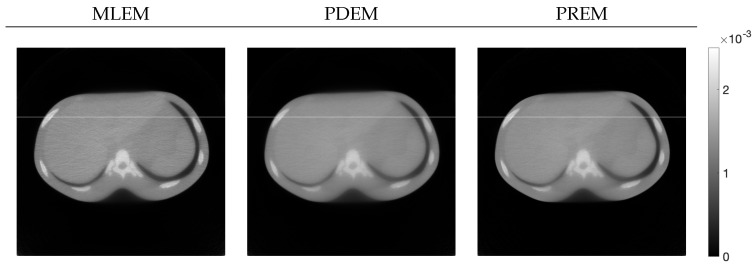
Images reconstructed from sinogram obtained from X-ray CT scanner by using MLEM, PDEM, and PREM (the white horizontal lines on the images indicate the positions of the density profiles in Figure 11).
